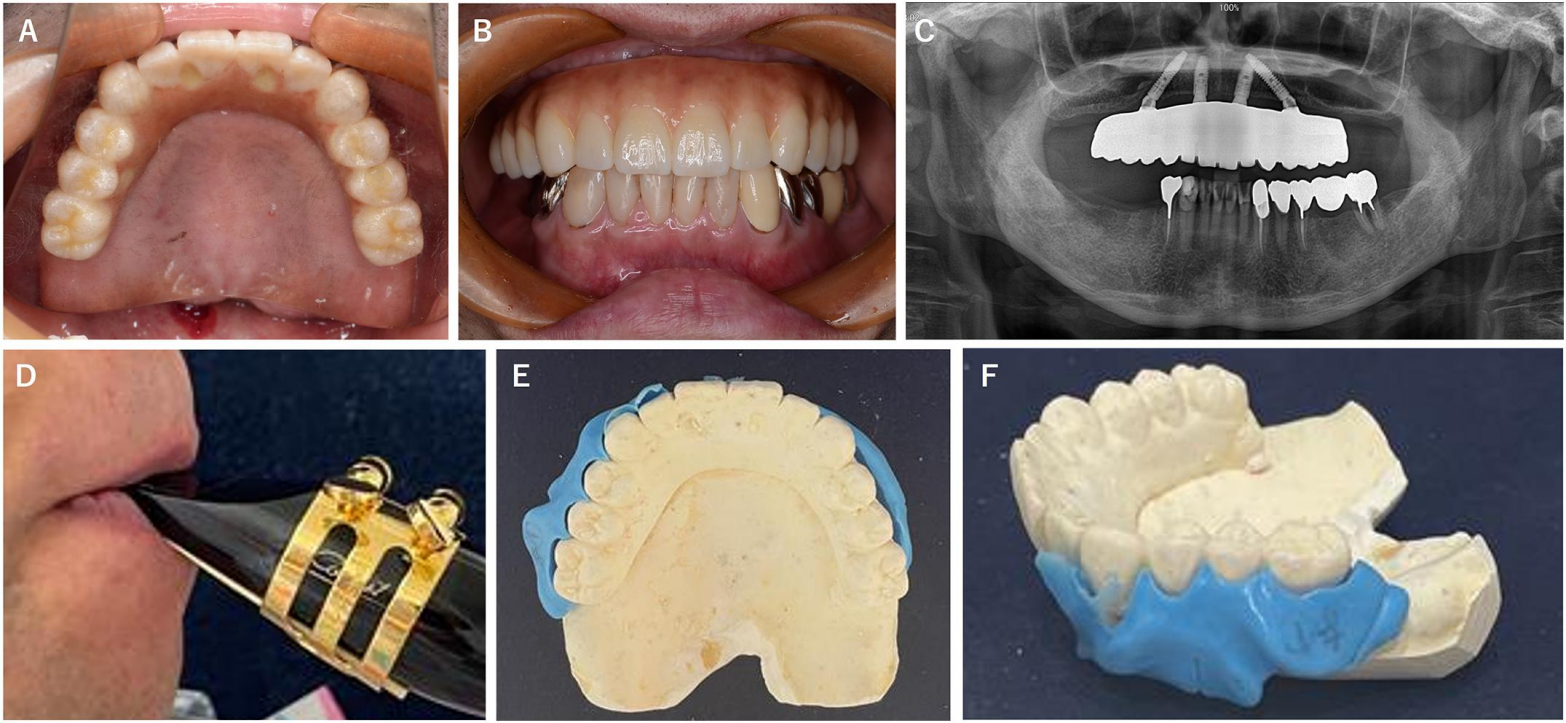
The patient's condition at the first visit. (A) Intraoral occlusal view. (B) Intraoral frontal view. (C) Panoramic x-ray of the upper and lower jaws. (D) The embouchure while the patient was playing the clarinet. (E) Lateral dental impression on the maxillary model, occlusal view. (F) Lateral dental impression on the maxillary model, lateral view.
